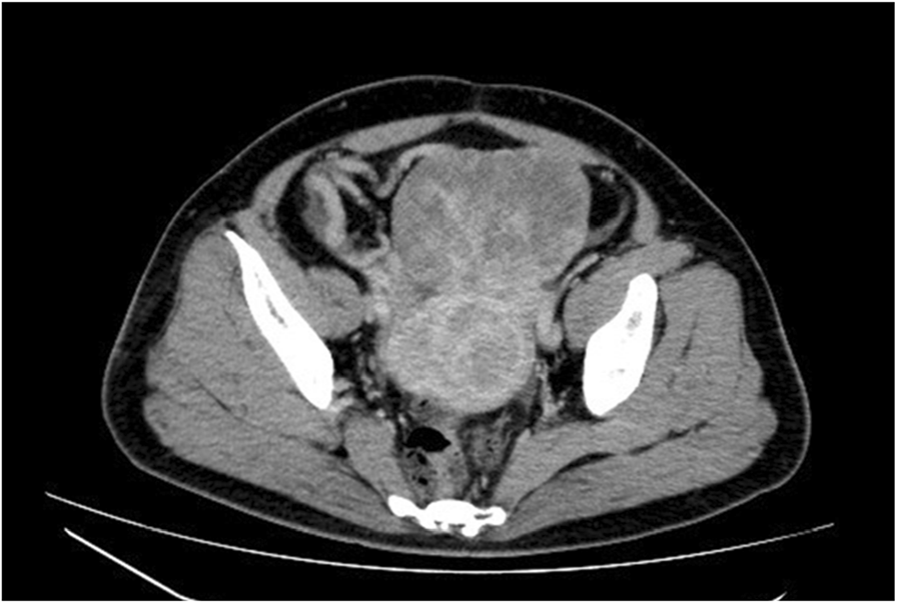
Contrast-enhanced computed tomography (CT) showing an irregularly enhanced solid mass measuring 10 × 8 cm in size in the cranial part of the uterine body, although the enhanced effect was lower. Lymph node enlargement suggestive of metastasis was not identified, and there were no metastases to other organs
